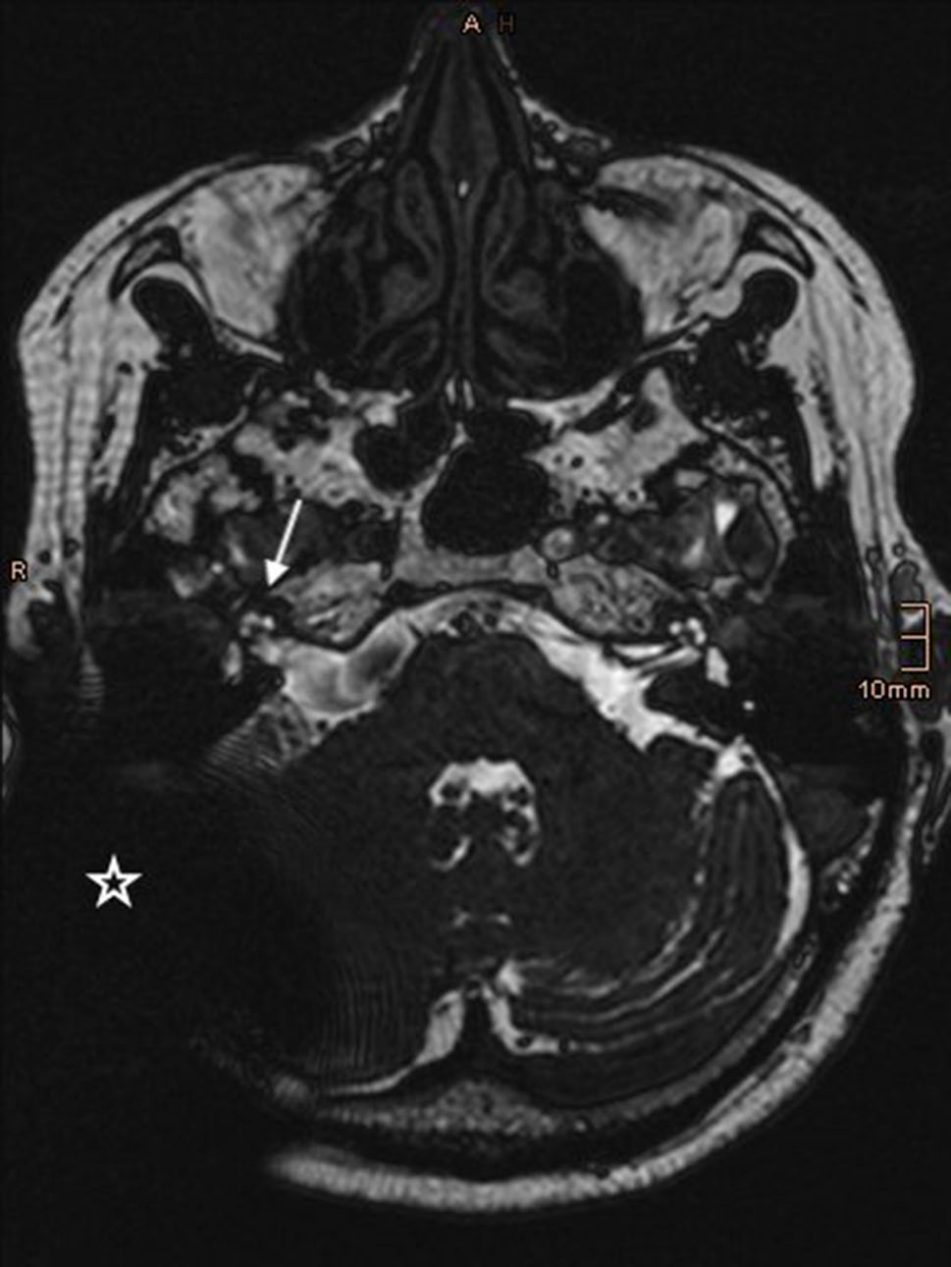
MRI CISS, axial, postoperative image. The arrow points at the cochlea, the star is placed in the large artifact created by the cochlea implant. This image was rated as Grade 2—good image quality
